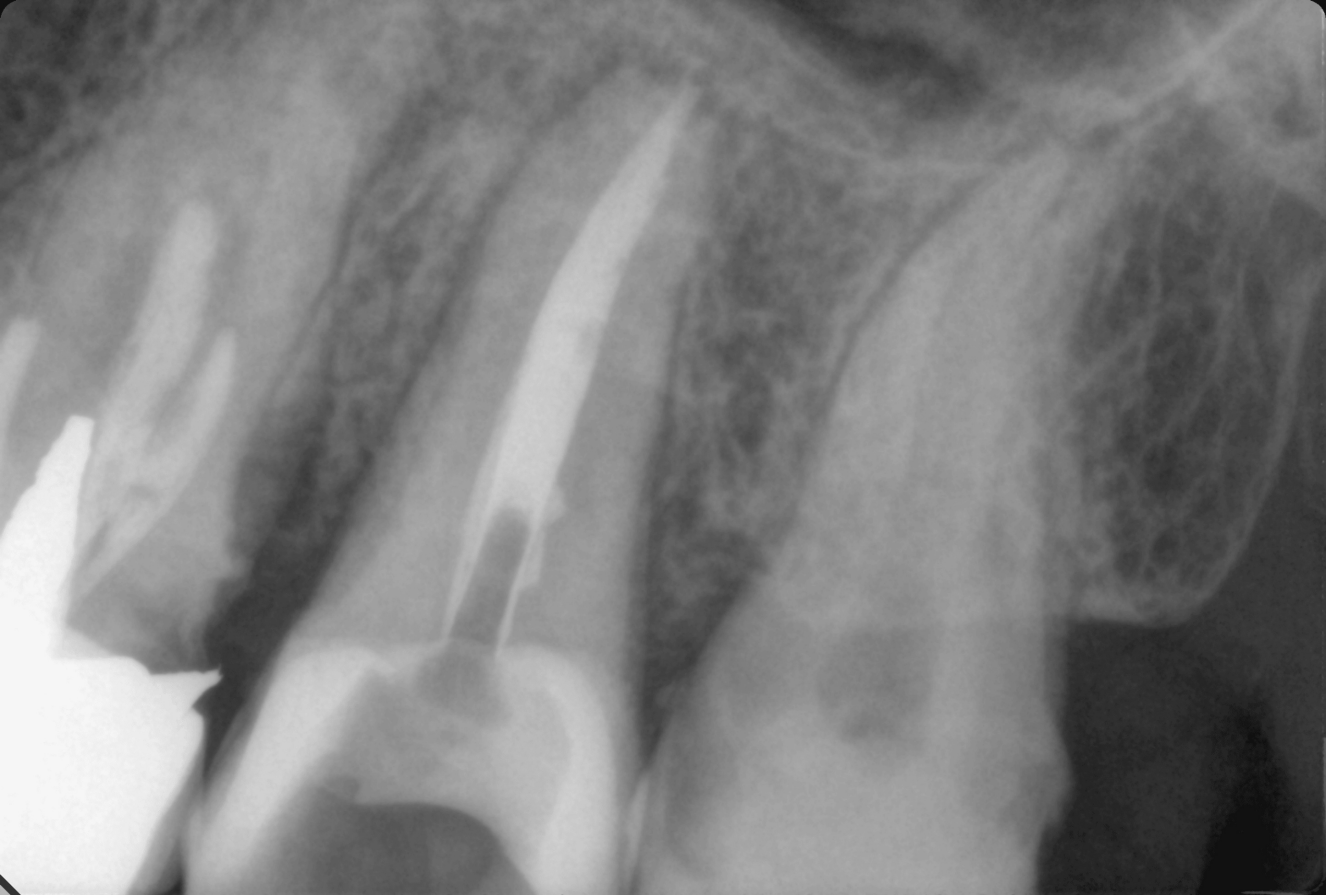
Postoperative radiograph of tooth 27
